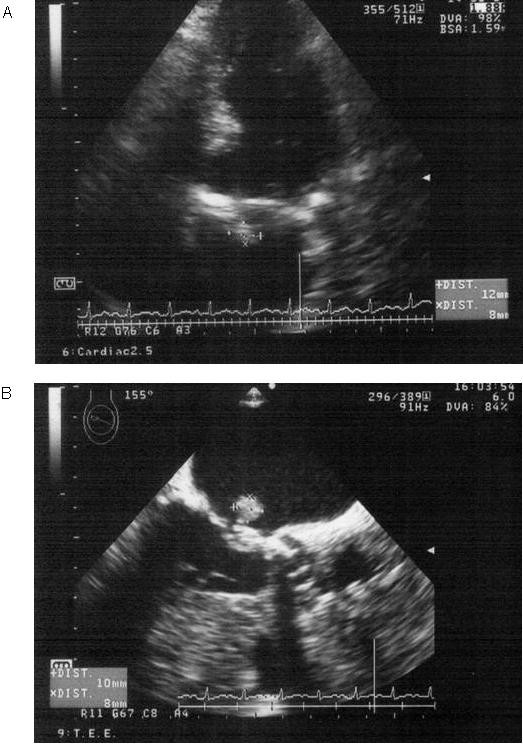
Transthoracic echocardiogram (A) and transesophageal echocardiogram (B) performed on day 13 revealed vegetation on the mitral valve.
